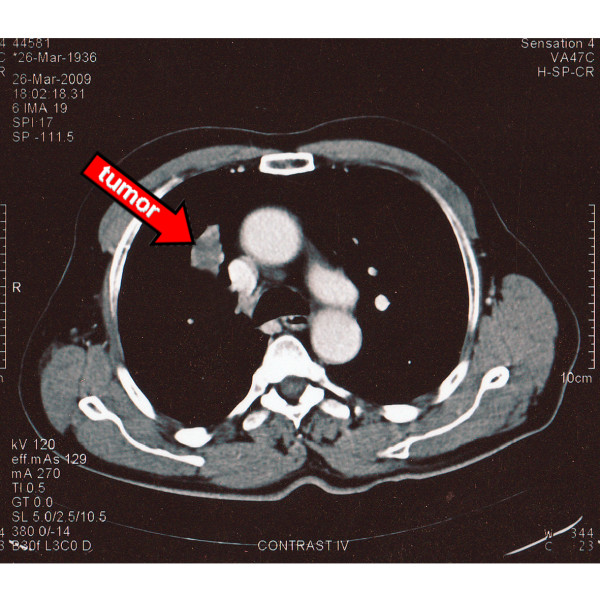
Chest computed tomography (CT) showed a tumor in the parahilar region of the right upper lobe.
